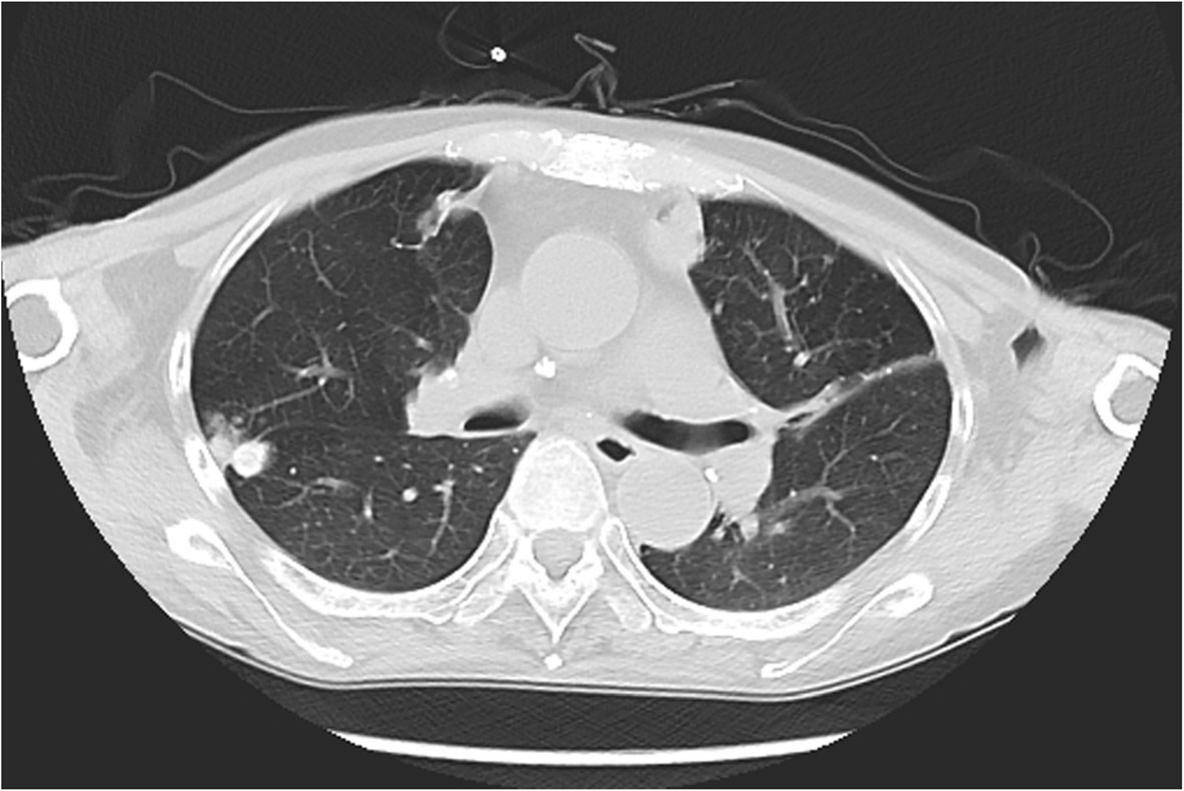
Chest computed tomography showed the bilateral patches in the lungs of the patient as opacities in the middle and lower lobes, multiple shadows of fibrotic streaks, high-density nodules, and mediastinal calcification of lymph nodes
